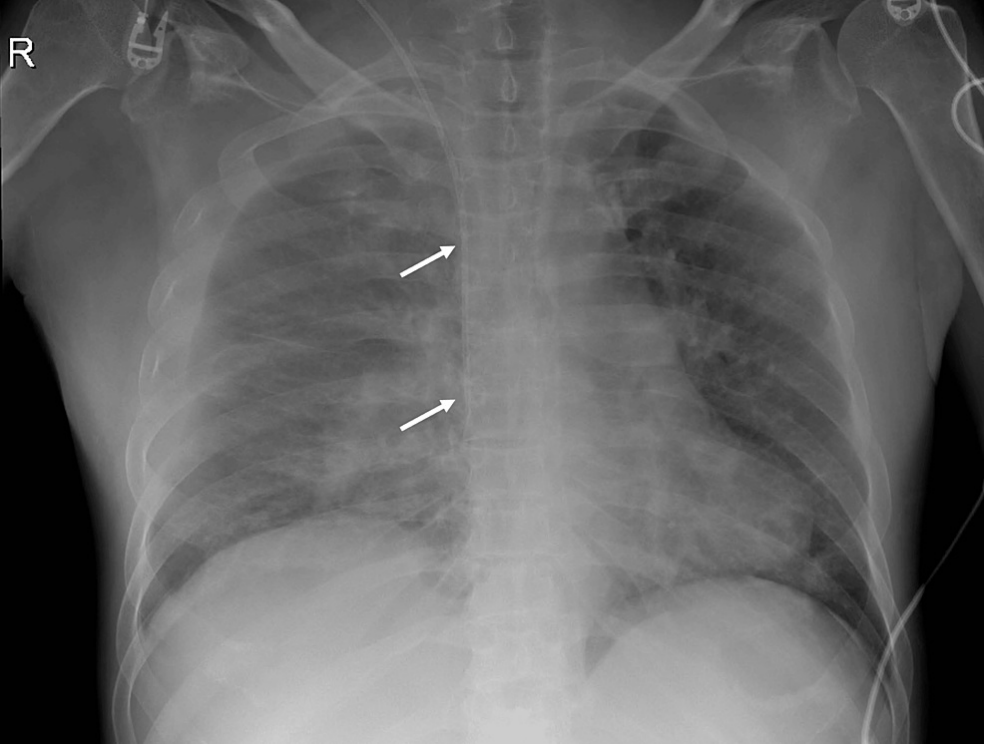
Anteroposterior CXR after CVC placement (white arrows show CVC location) CXR, chest X-ray; CVC, central venous catheter
